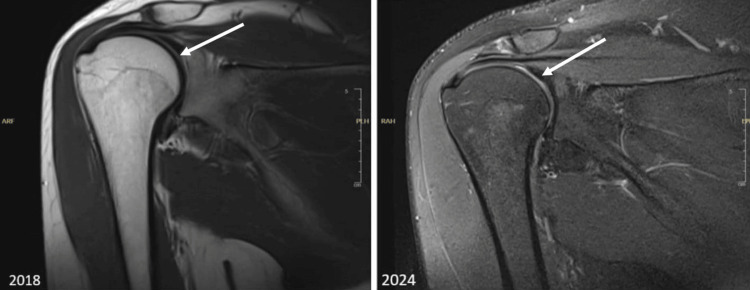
Right shoulder MRI comparison 2018 and 2024 showed no rotator cuff tendinopathy and minimal subacromial subdeltoid bursitis and minimal supraspinatus tendinosis, and no rotator cuff tear.
